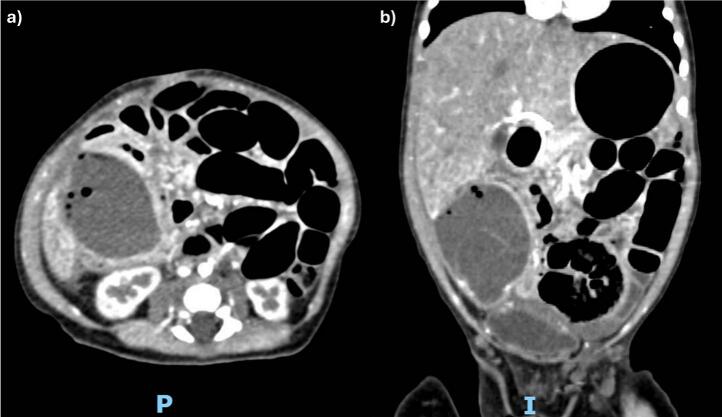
Axial (a) and coronal (b) abdominal CT scan with intravenous contrast showing a right-sided heterogeneous abdominal mass filled with air and liquid, adjacent to the liver and small bowel.
